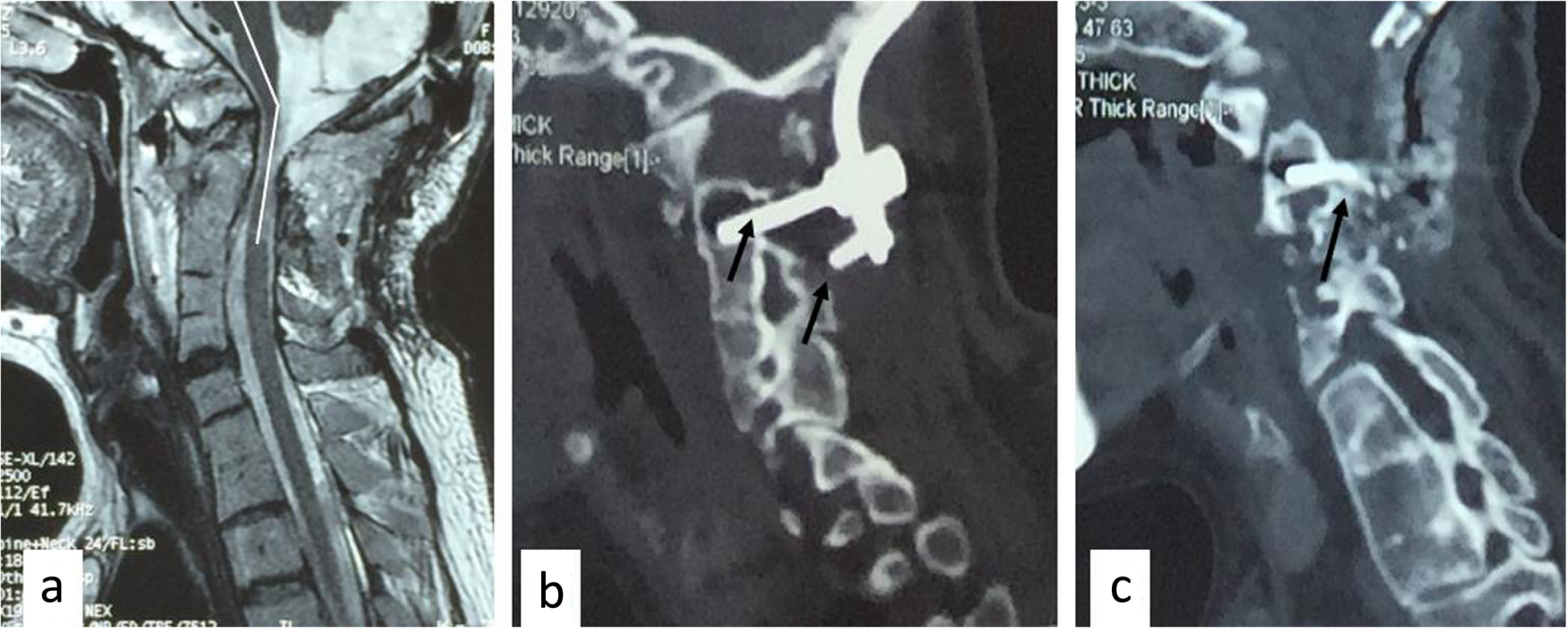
CT and MRI scan after revision surgery. a The compression of the ventral side of the brainstem and the upper cervical cord was significantly relieved, and the cervicomedullary angle returned to normal. b, c CT sagittal reconstruction image showed screws loosening and pull-out
